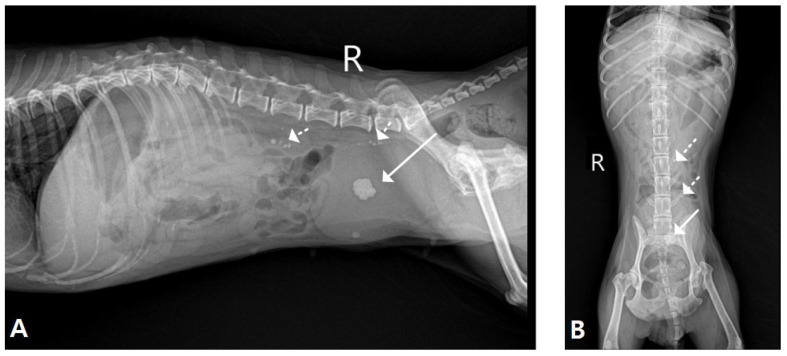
Radiographic findings of the urinary tract. (A) Lateral radiographic view showing radiopaque uroliths within the urinary bladder (arrow with line) and distension of the urinary bladder. Additional radiopaque calculi were observed within the kidneys and ureters (arrow with dotted line). (B) Ventrodorsal radiographic view demonstrating multiple radiopaque uroliths within the urinary bladder (arrow with line) and calculi distributed along the ureters and kidneys (arrow with dotted line).
